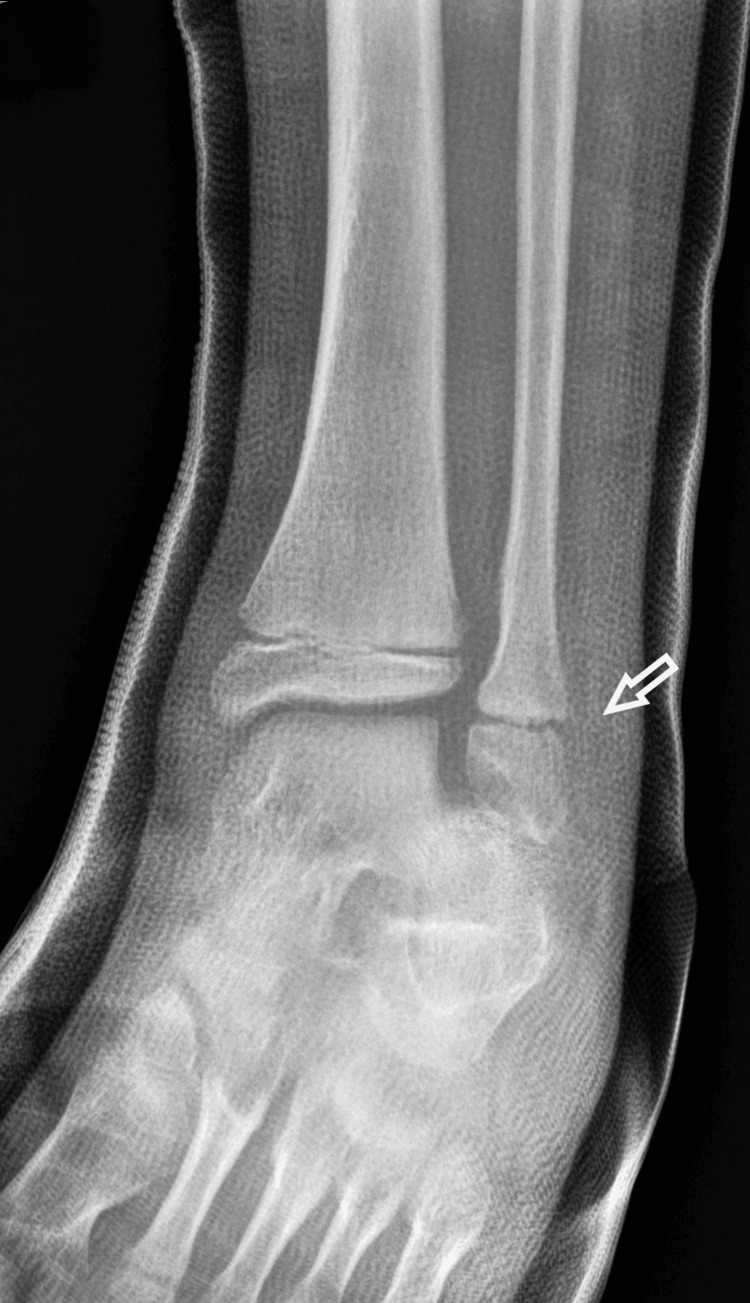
AP X-ray, after reduction of the fracture and immobilization in a cast. The arrow is pointing the normal alignment of the distal fibular epiphysis.
